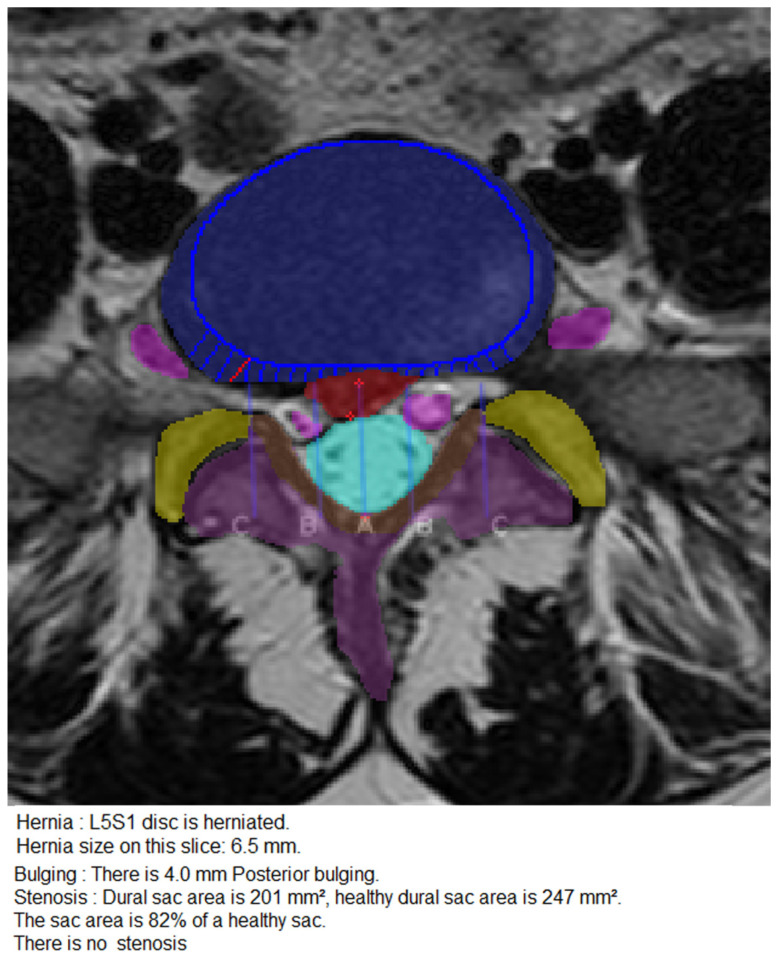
Measurement and classification of disc herniation, disc bulging, nerve root compression and spinal canal stenosis. T2-weighted, axial slice through the segment L5/S1 at disc level showing disc herniation, disc bulging, no nerve root compression and no spinal canal stenosis. The projected contour of the vertebral body adjacent to the disc is represented by the rounded blue line, the intervertebral disc is represented by the blue area. The red area represents herniated disc material, the distance between the red crosses is measured 6.5 mm and is therefore correctly classified as disc herniation. The blue lines and the single red line on the right side of the disc perpendicular to the projection of the contour of the vertebral body represent measurements of the disc exceeding the boundaries of the adjacent vertebral bodies, correctly reported as 4 mm bulging. The nerve roots (pink) have no contact to either the herniated or the bulging parts of the disc, therefore nerve root compression was correctly classified as absent. The light blue area represents the dural sac of 201 mm^2^. There was no spinal canal stenosis reported.
